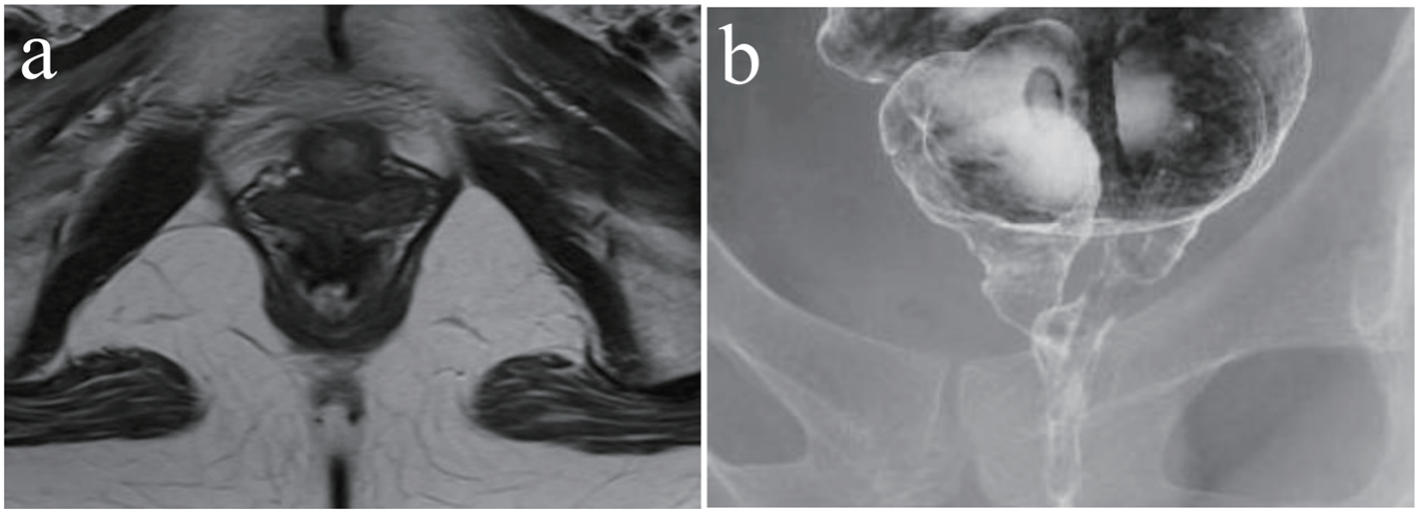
(a) MRI. (b) Barium-enema study. Both examinations are inspected from the start of therapy 1 year later, and confirmed rectovaginal fistula closing.
